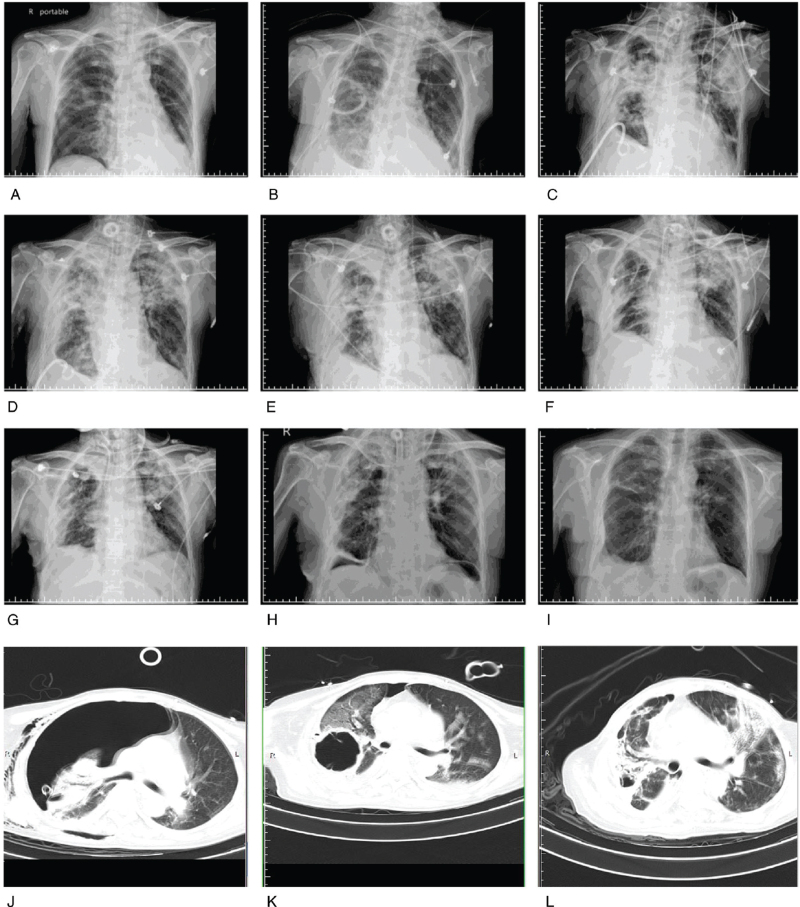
The patient's serial chest radiographs. Day of hospitalization: (A) D 24, (B) D 29, (C) D 36, (D) D 45, (E) D 49, (F) D 73, (G) D 80, (H) D 104, and (I) 28 days after discharge. Chest computed tomography scan on D 25 (J), D 31 (K), and D 57 (L).
